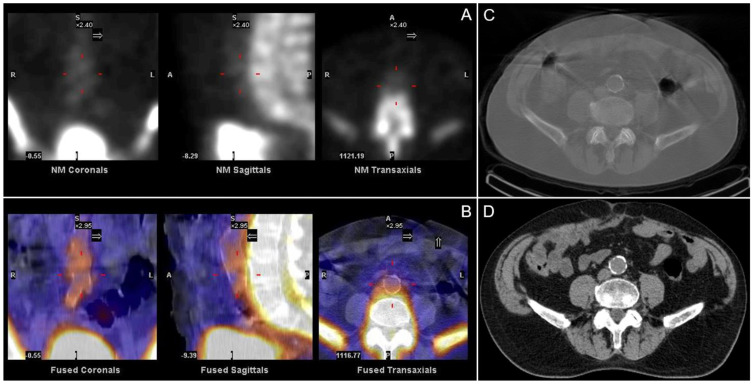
SPECT/ (A) and co-registered SPECT/CT (B) to the non-anatomical CT (C) of the spine using ^99m^Tc MDP shows typical blurring of the anterior vertebral body that corresponds to calcification of the descending aorta on the CT (D).
